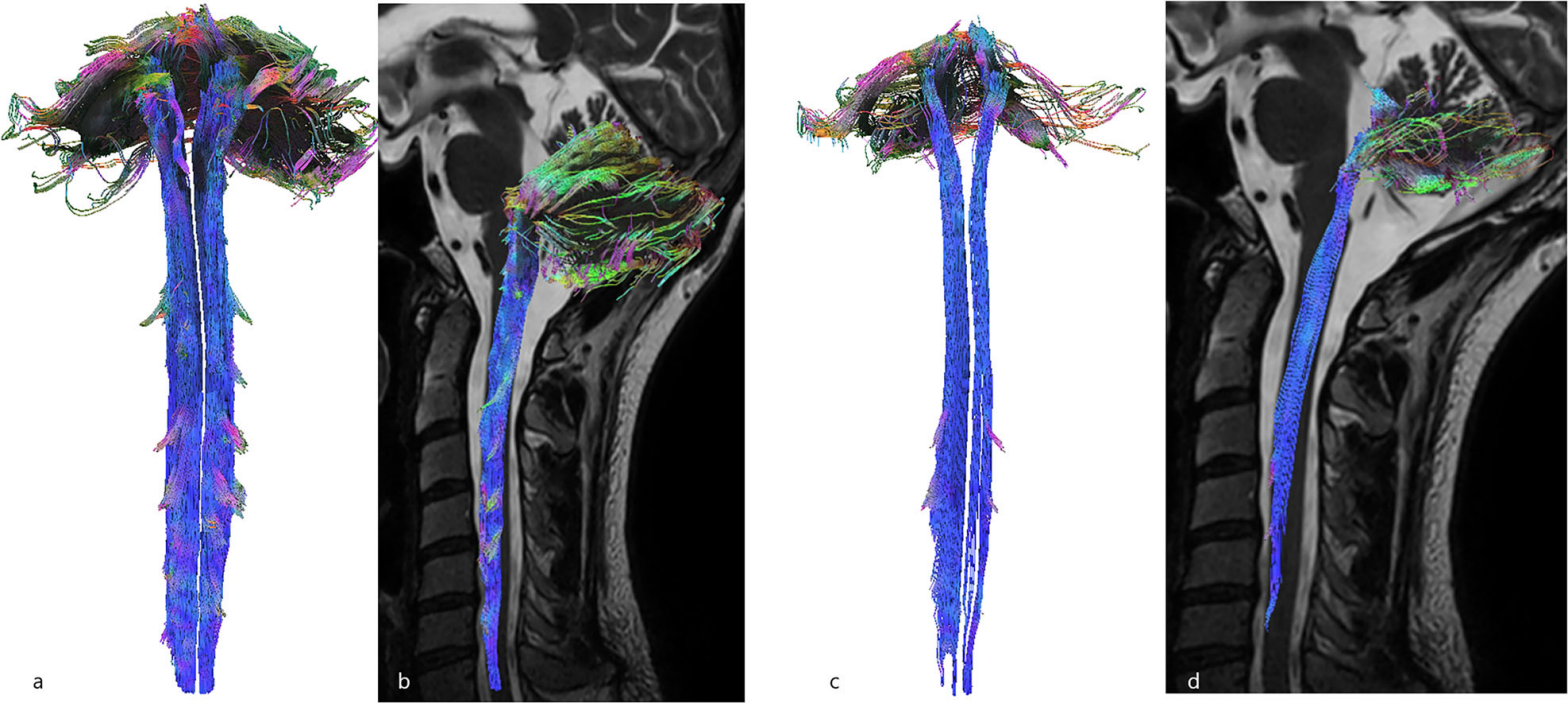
3D-tractography rendering of the spinocerebellar tracts. a Coronal view of the dorsal spinocerebellar tract. b Sagittal view of the dorsal spinocerebellar tract merged on sagittal T2-weighted images. c Coronal view of the ventral spinocerebellar tract. d Sagittal view of the ventral spinocerebellar tract merged on sagittal T2-weighted images
